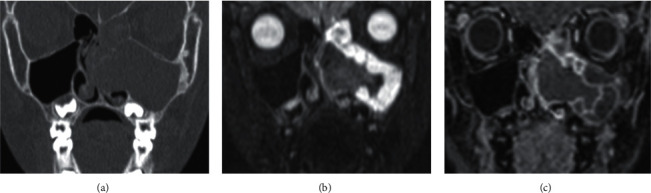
Fungal sinusitis in 7-year-old male with symptoms of nasal obstruction. (a) Coronal unenhanced CT demonstrates left ostiomeatal pattern opacification with maxillary mural osteitis plus expansion of maxillary antrum. This appearance is equivocal and could represent chronic rhinosinusitis, fungal sinusitis, or tumour. However, low signal centrally on (b) coronal STIR, surrounded by peripherally enhancing thickened mucosa on (c) coronal contrast-enhanced T1W, is consistent with a left maxillary into middle meatal mycetoma and maxillary sinusitis.
